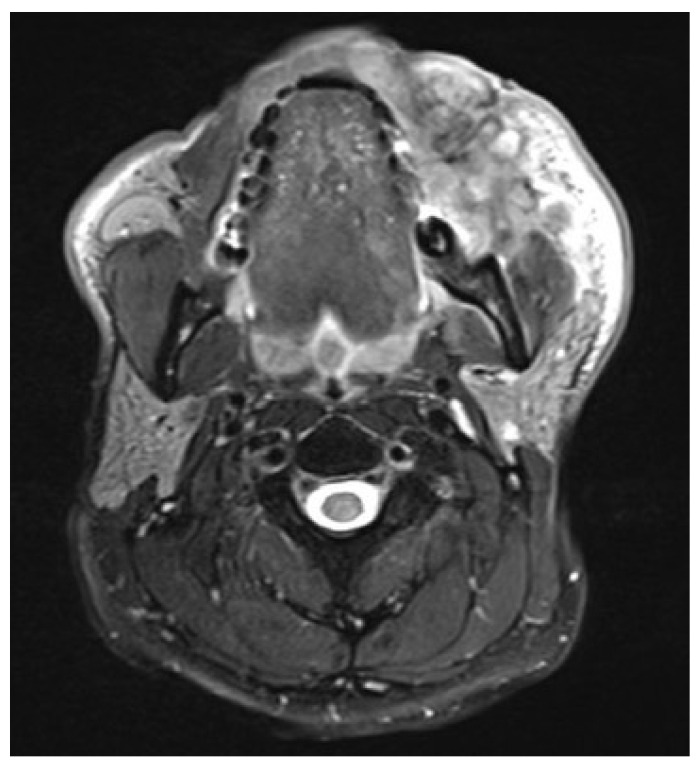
Case 5—Preoperative staging T2W magnetic resonance imaging (axial view).
